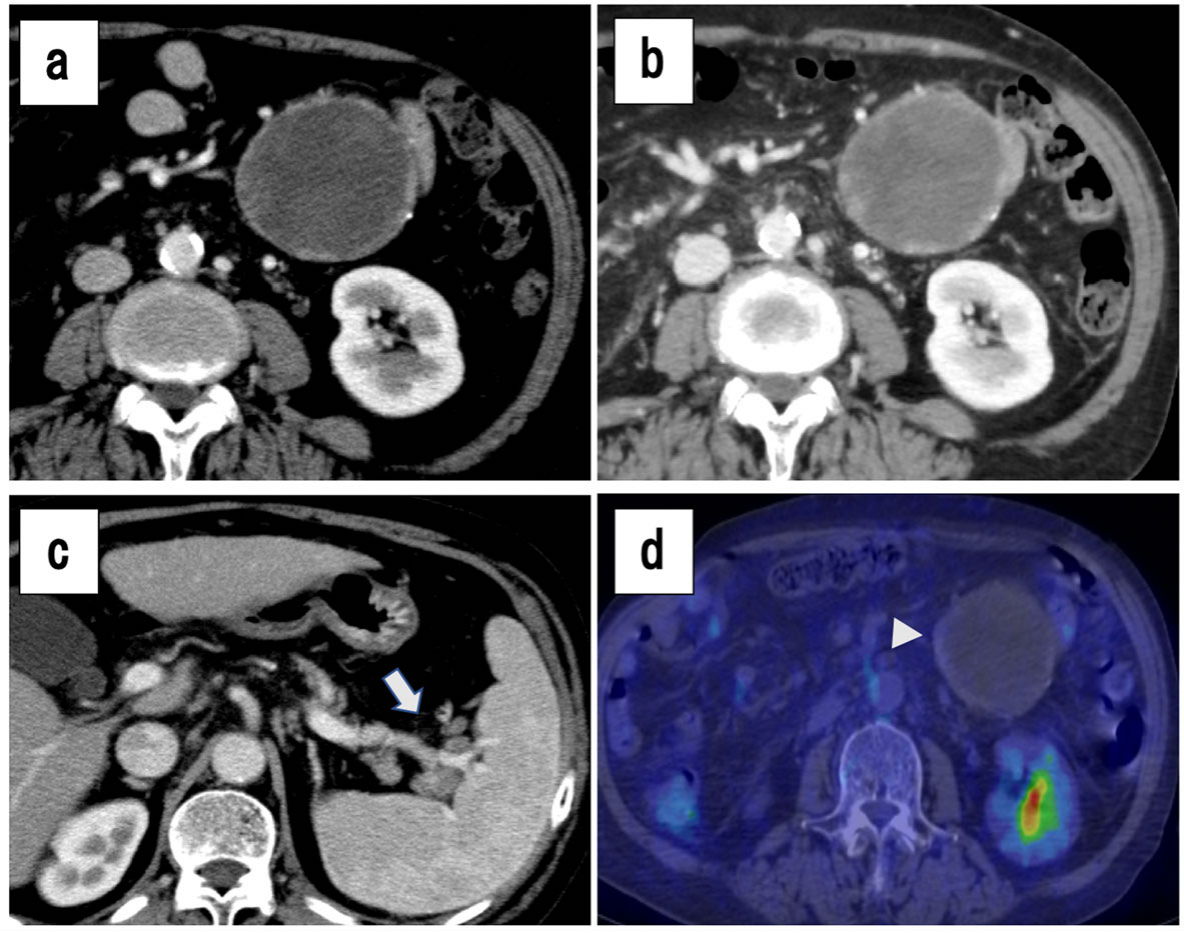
Abdominal contrast-enhanced computed tomography (CT) findings (a–c). a A well-defined, mixed-density, round tumor with a maximum diameter of 77 mm, adjacent to the upper jejunum. b The tumor was accompanied by calcification at part of the border. c Diffuse lymphadenopathy was detected at the paraaortic, splenic artery region, and splenic hilum area (arrow). d (18)F-FDG PET-CT showed slight accumulation (SUVmax 1.5–2.0) along the tumor border, corresponding to the calcified portion (arrowhead)
